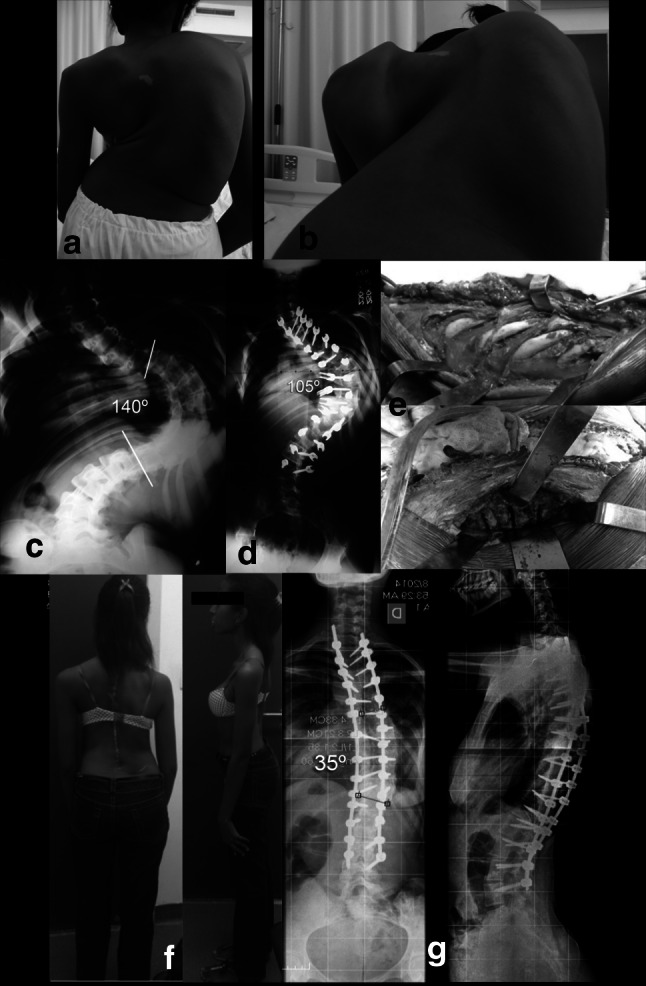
20-year-old patient with neglected AIS—severe curve of 140° treated with staged procedures. a, b Preoperative clinical pictures. c Preoperative PA radiograph. d Radiograph after posterior release and instrumentation and 2 weeks of traction. e Intraoperative pictures of PEISR technique. f, g Postoperative clinical and radiographical correction
